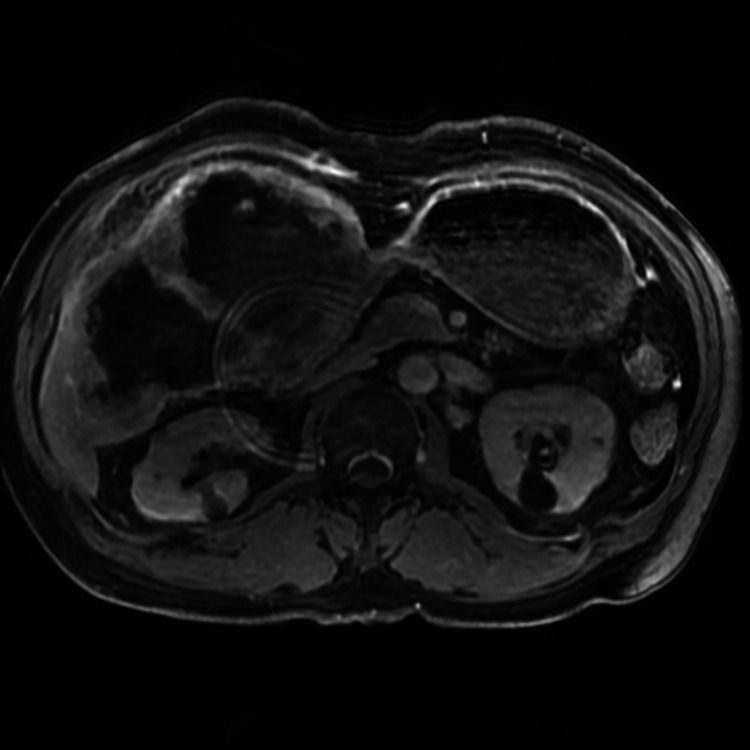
MRI Liver (axial view) showing large, heterogenous and extensively necrotic mass invading the liver with peripheral enhancement arising from the gallbladder. MRI: magnetic resonance imaging.
